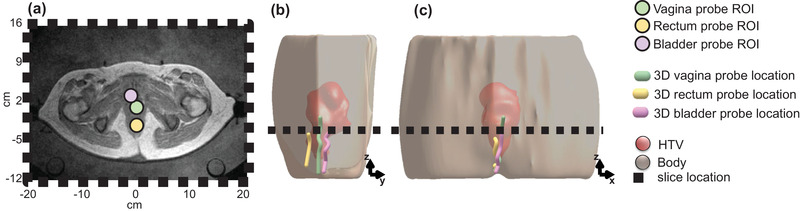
Axial T2‐weighted MR image (a) of the pelvic region is shown along with the Bowman probes ROIs: vagina, rectum, and bladder. The location and path of the probes in the 3D model is shown in the sagittal (b) and coronal (c) view. The dotted line represents the axial location of the T2‐weighted MR image
